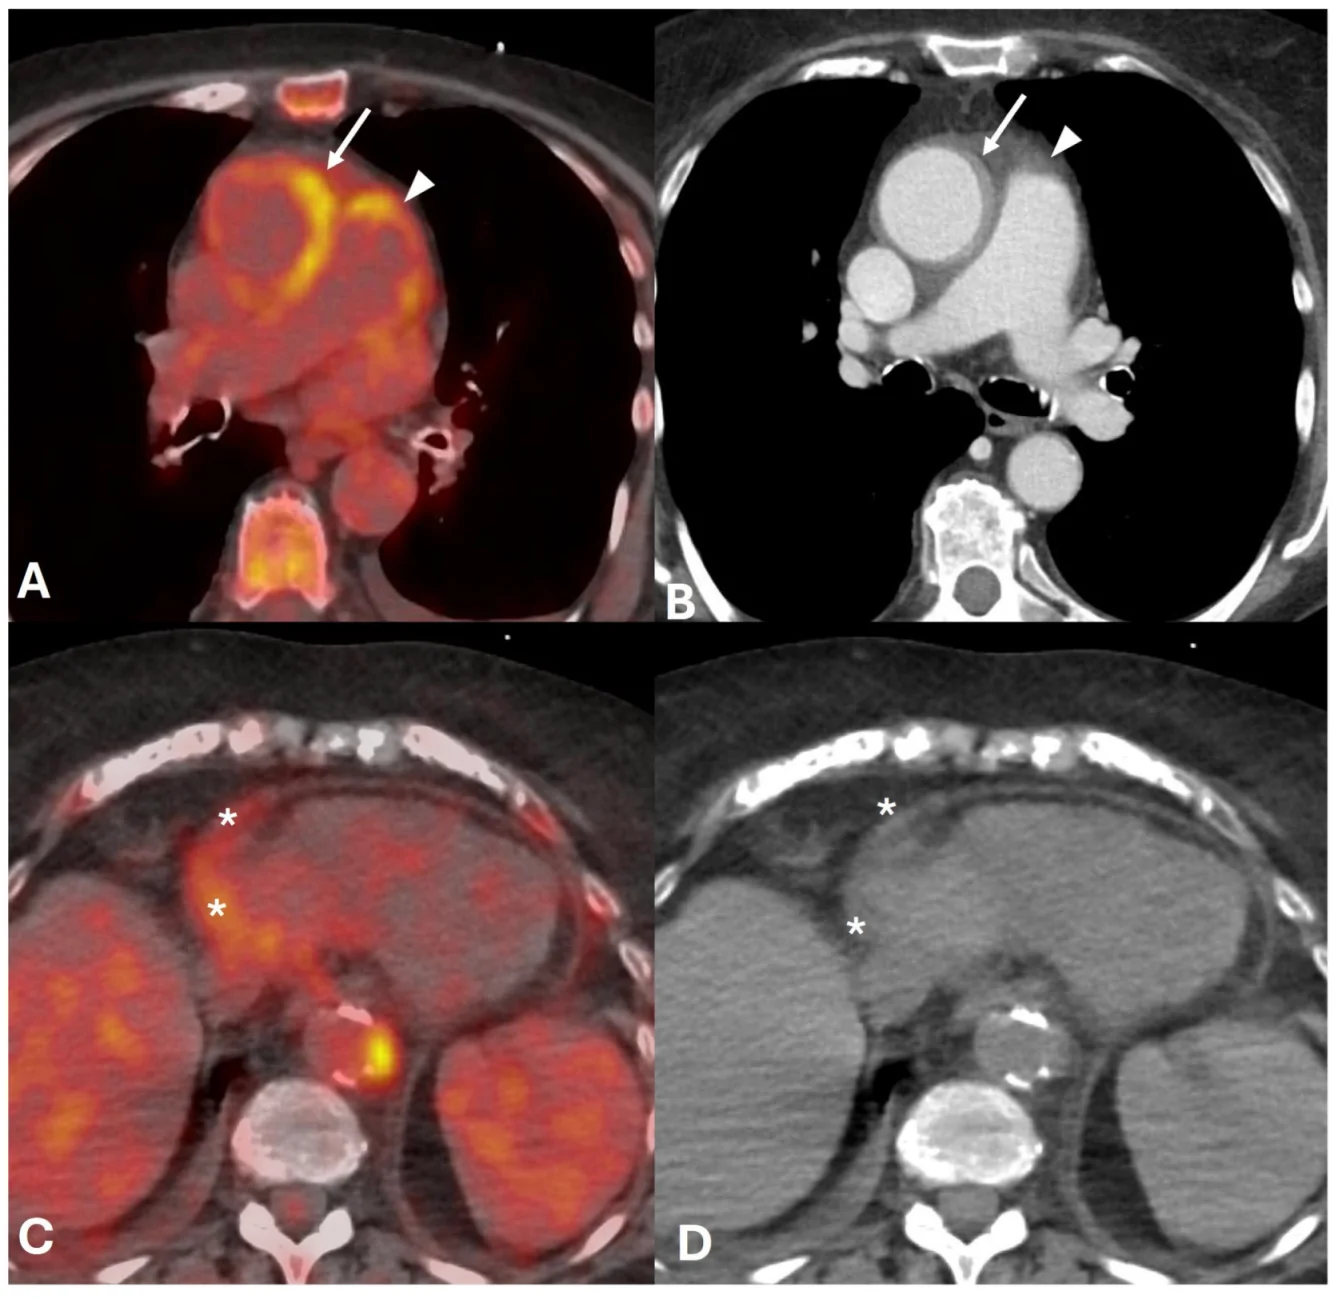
An example of known Bechets disease presenting with active vasculitis and pericarditis. Axial fused images of F-18 FDG PET/CT (A,C), axial image of CT chest with intravenous contrast (B) and axial image of CT chest without contrast (D) showing tracer avid concentric mural thickening to the ascending aorta (arrows) and to a lesser extent main pulmonary artery (arrowheads) as well as tracer avid pericardial thickening (*).
